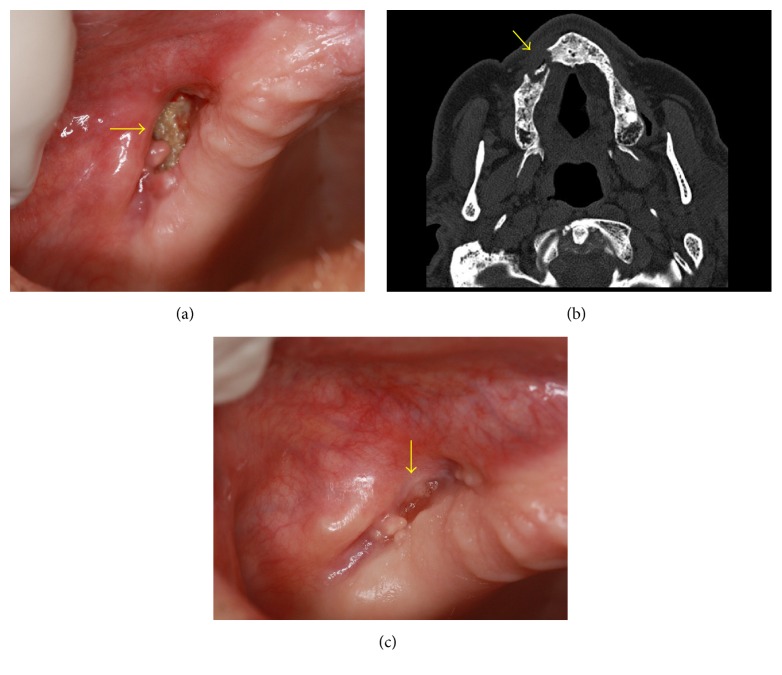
Clinical aspect of bone exposure measuring approximately 1.5 cm at vestibular sulcus of right maxilla (a). CT showing bone lysis and necrotic bone sequestrum at maxilla with oral antral communication risk (b). Clinical aspect of vestibular sulcus mucosa totally recovered after 37 sessions of LLLT and PDT (c).
